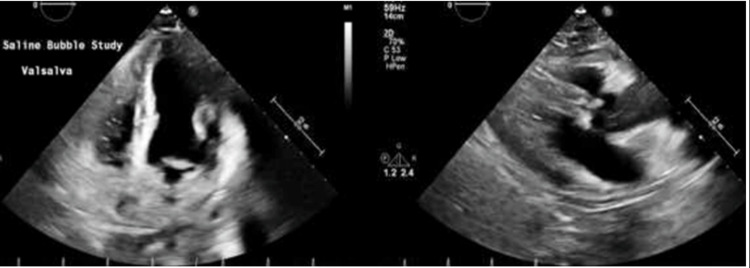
Transthoracic echocardiogram on February 17, 2022 Conclusion: LVEF is 70%, grade I mild diastolic dysfunction, no evidence of patent foramen ovale by agitated saline contrast, mildly elevated RV systolic pressure, and no significant pericardial effusion.
